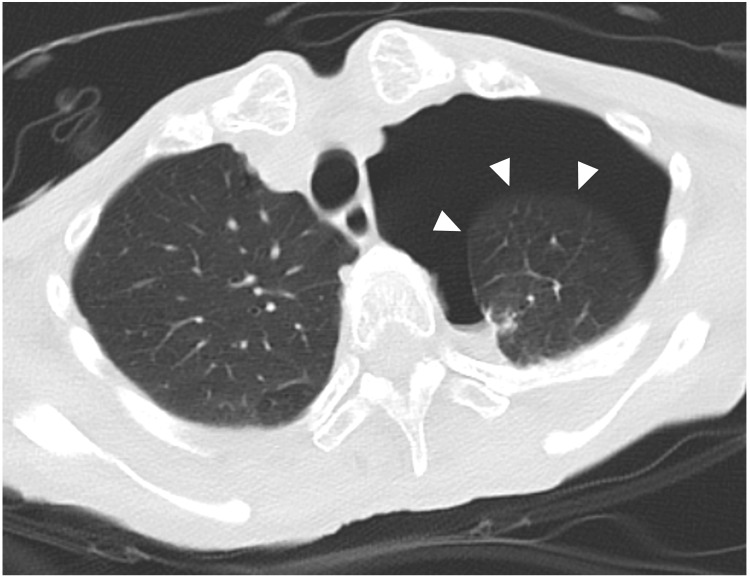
Axial CT on postoperative day one (Case 6) Marked progression of the left-sided pneumothorax is demonstrated (white arrowheads). Chest tube placement was performed following this examination. CT, computed tomography
